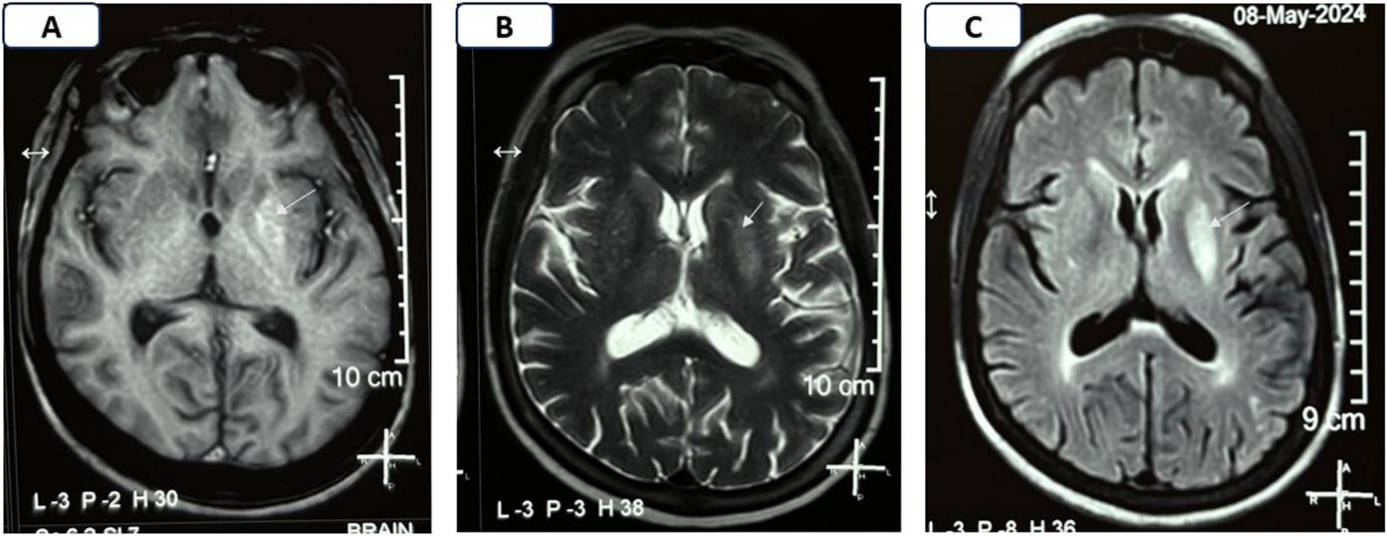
Magnetic resonance imaging of the brain done at presentation showing hyperintensity on T1, characteristic of diabetic striatopathy (A); hyperintensity on T2 (B); and fluid-attenuated inversion recovery (C) sequences in the left lentiform nucleus (white arrows).
